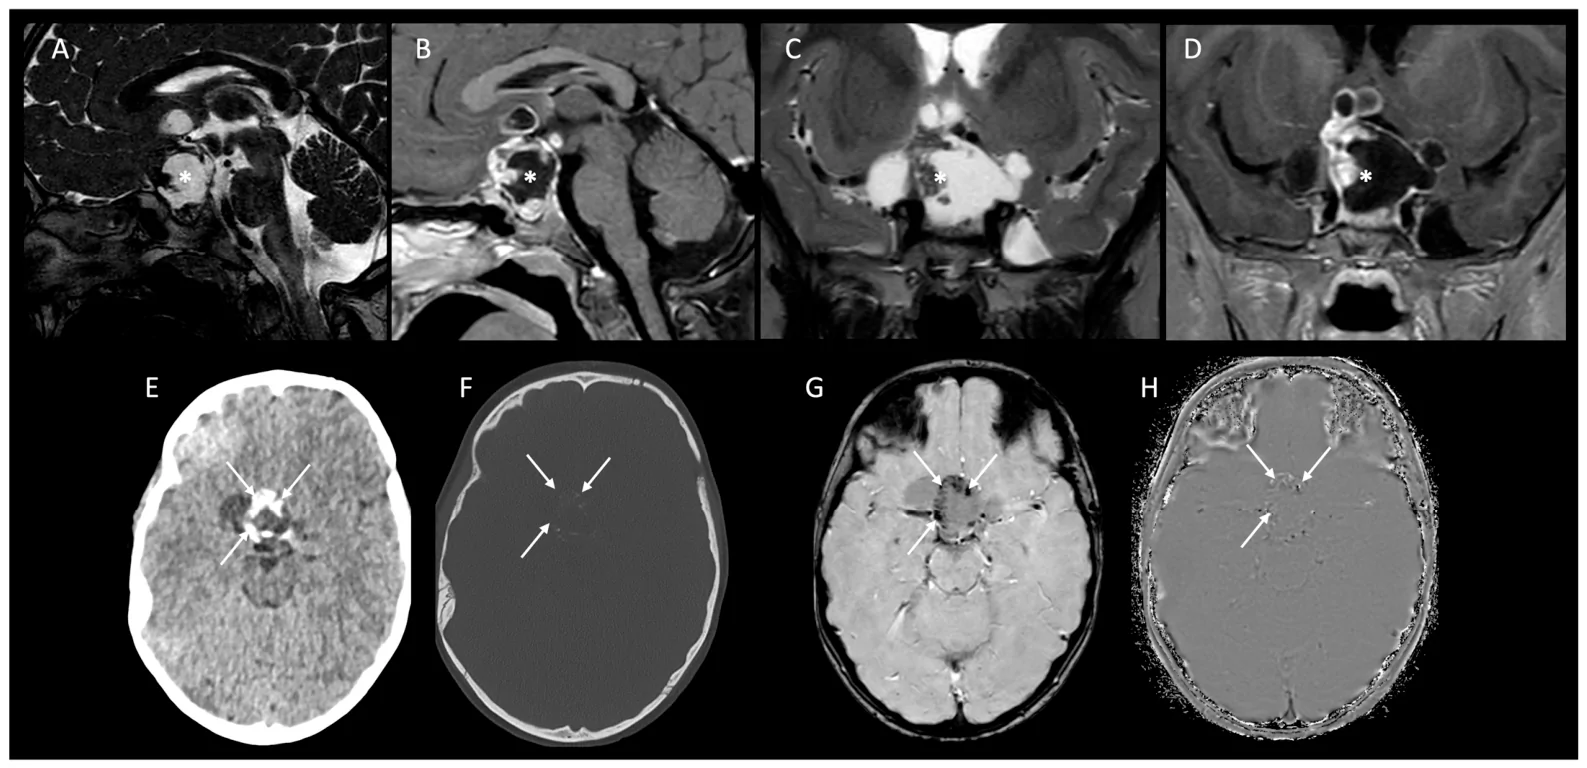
ACP. MRI and CT studies of a 5-year-old boy with headache and vomiting. (A) Sagittal bSSFP, (B) sagittal and (C) coronal contrast-enhanced T1, (D) coronal T2, (E) axial CT, (F) axial bone CT, (G) axial magnitude and (H) phase maps of SWI. Multiloculated cystic lesions involving the sellar and suprasellar cistern and bilateral anterior and middle cranial fossae (asterisks). The lesion shows multiple calcifications (arrows), which are easily identified on CT but also well seen on SWI and can be differentiated from hemorrhage (calcifications are black on magnitude and white on phase maps of SWI in the “right-handed” system, as here).
